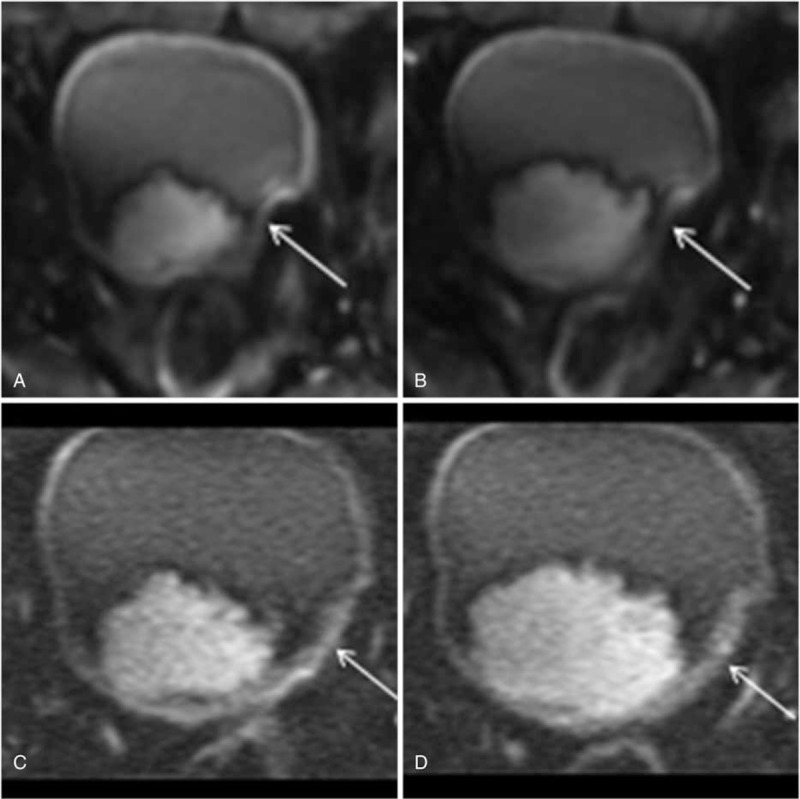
A 64-year-old man with stage pT1 papillary urothelial carcinoma. A, B, The axial fFOV DW MRI shows a severe magnetic susceptibility artifact at the junction of the bladder and rectum (arrow). C, D, The axial rFOV DW MRI demonstrates a significantly reduced artifact in this location (arrow). DW = diffusion-weighted, fFOV = full field of view, MRI = magnetic resonance imaging, rFOV = reduced field of view.
